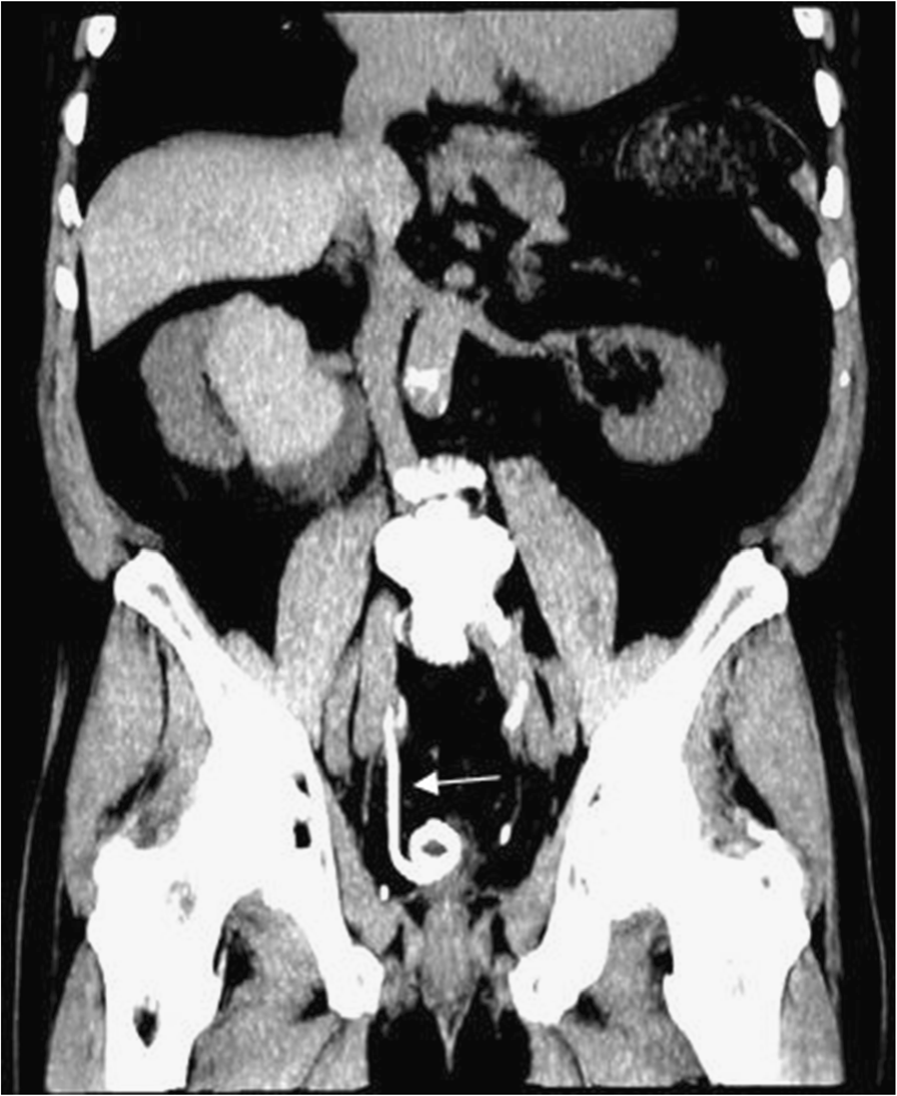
Four days later computed tomography scan. This computed tomography scan obtained 4 days after the rupture shows the cyst with residual contrast, confirming the communication with the pelvicalyceal system, and the placement of the double-J catheter for treatment (arrow).
